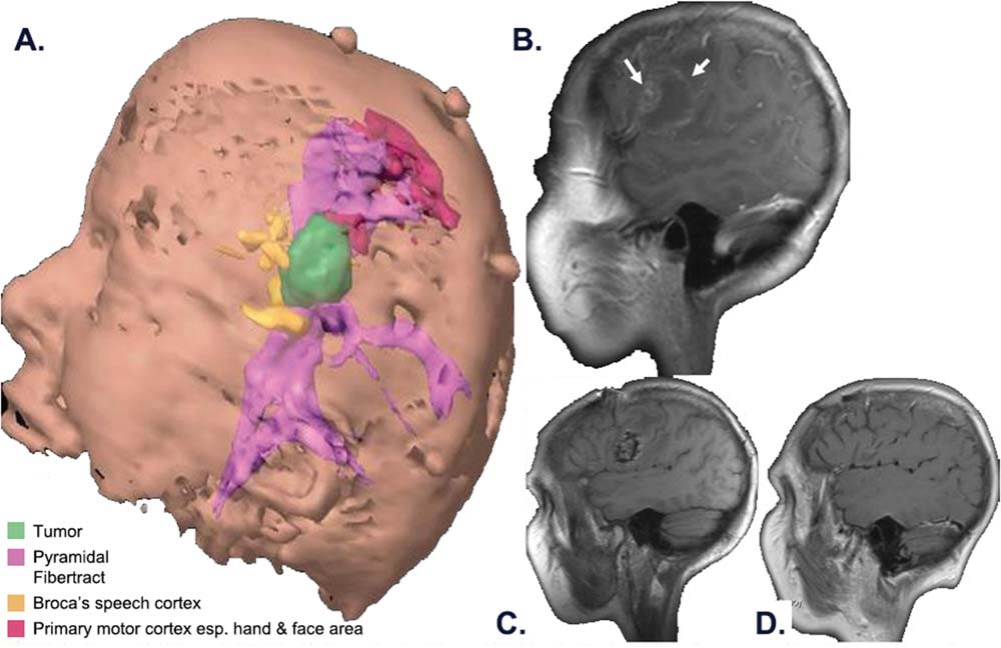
Preoperative and postoperative magnetic resonance images (MRIs) are shown. A patient with metastatic melanoma developed aphasia and facial weakness. (A) The 3-dimensional preoperative model reveals the tumor (green), the primary motor cortex (red) and arcuate fasciculus (purple), the Broca speech area (yellow), and the corticospinal tract (purple). (B) This preoperative sagittal MRI reveals a single hemorrhagic metastasis (arrows) in the left frontal lobe near the primary motor and the Broca speech areas. (C) This postoperative MRI reveals resection of the tumor and intracavitary placement of the carmustine wafers. Speech, motor, and neurocognitive function returned postoperatively. (D) A follow-up scan shows no tumor recurrence at 34 months.
